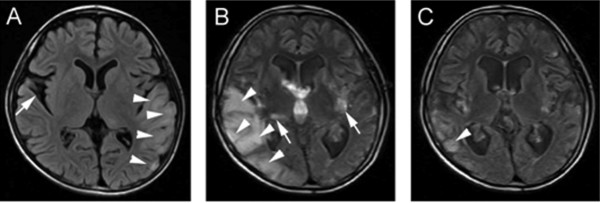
Brain MRI (FLAIR, fluid-attenuated inversion recovery) of patient 1: (A) taken one year before admission;: Abnormal signals in the left occipital, parietal, and temporal lobes (arrowheads) and a cyst (arrow) in the right parietal area. (B) Abnormal MRI signals shifted to the right side (arrowheads) at admission from previous left side. Symmetrical lesions at the lenticular nuclei (arrow) were also observed. (C) These abnormalities were dramatically decreased (arrowheads) after 30 days cocktail treatment in hospital.
